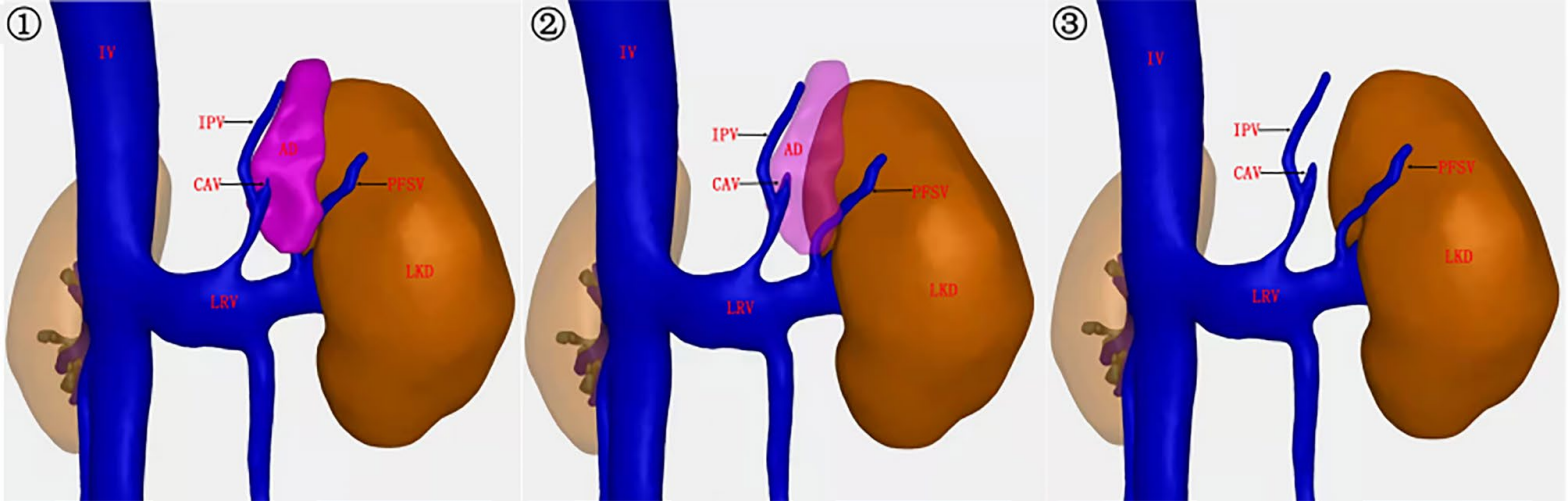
CT three-dimensional (3D) reconstruction stereoscopically showing the location and shape of PFSV. By changing the transparency of the adrenal gland, we could easily identify that the PFSV travels upward along the lateral border of the left AD into the direction of the diaphragm and drains down into the left RV ultimately. *IPV* inferior phrenic vein, *LKD* left kidney, *LRV* left renal vein.
